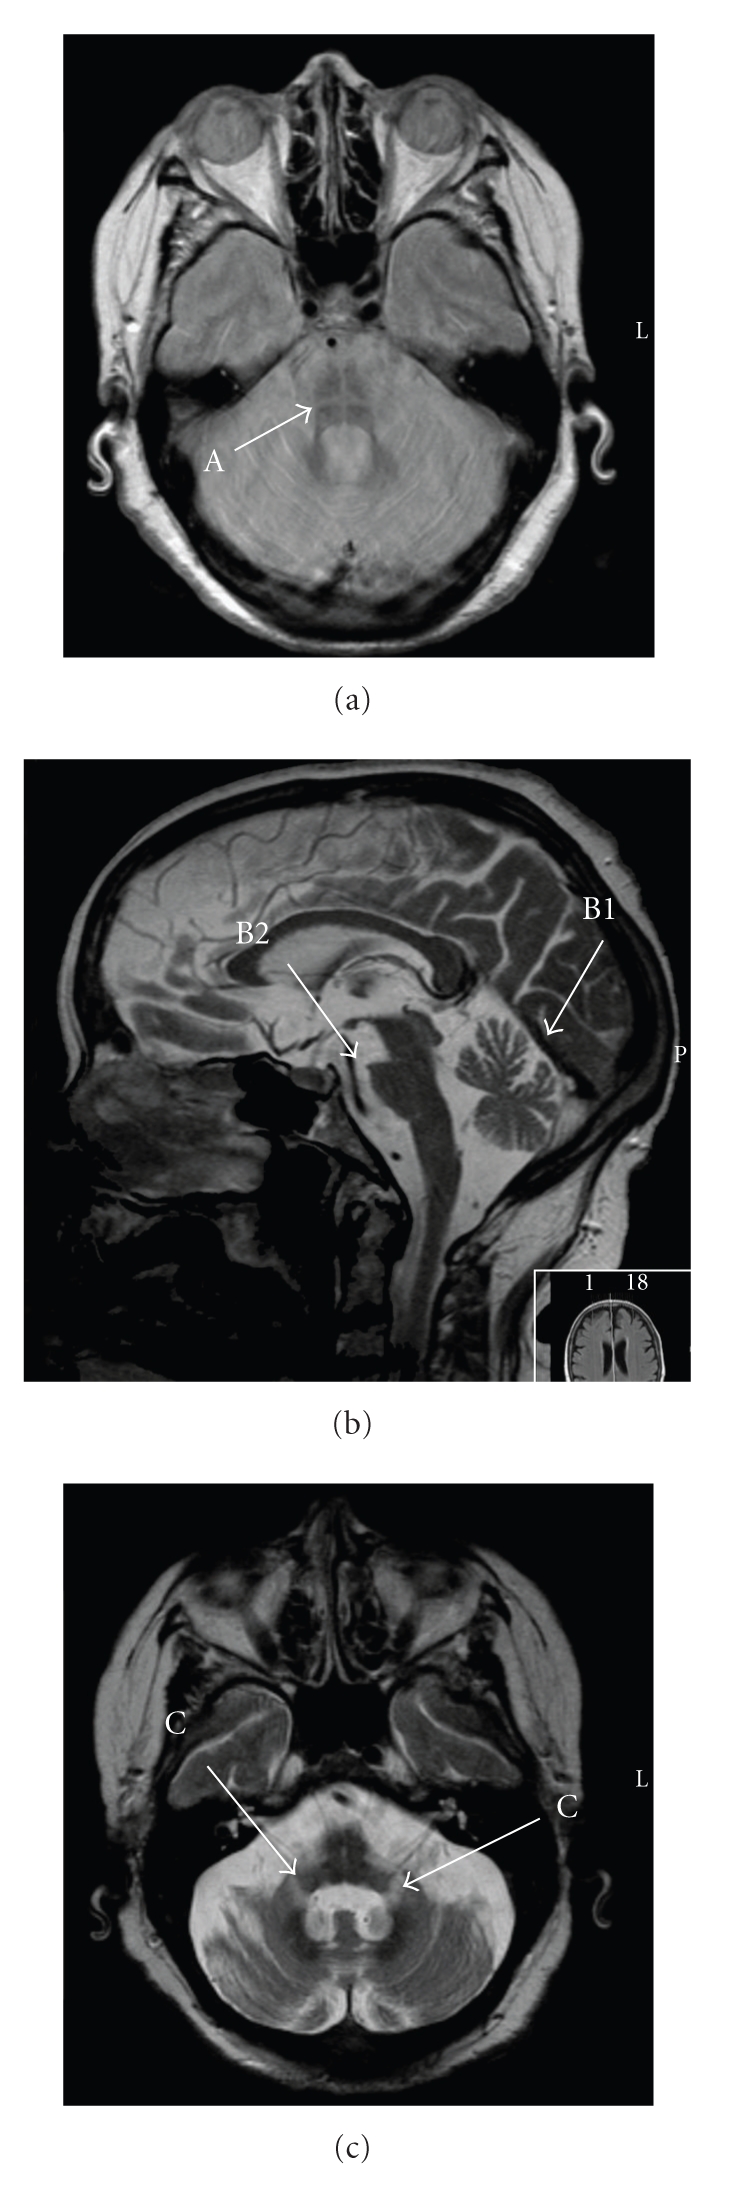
MRI imaging's showing the reported alterations. (A) Hot cross sign. (B1) cerebellar and (B2) pons atrophy. (C) Atrophy of middle cerebellum peduncles.
